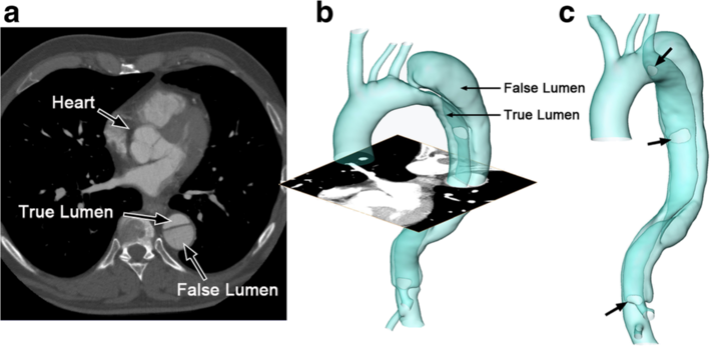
The reconstructed surface of the aortic dissection. (a) is one axial slice of the CT scan; (b) is the reconstructed surface of the aortic dissection; (c) shows the positions of the entries along the flap (indicated by arrows).
